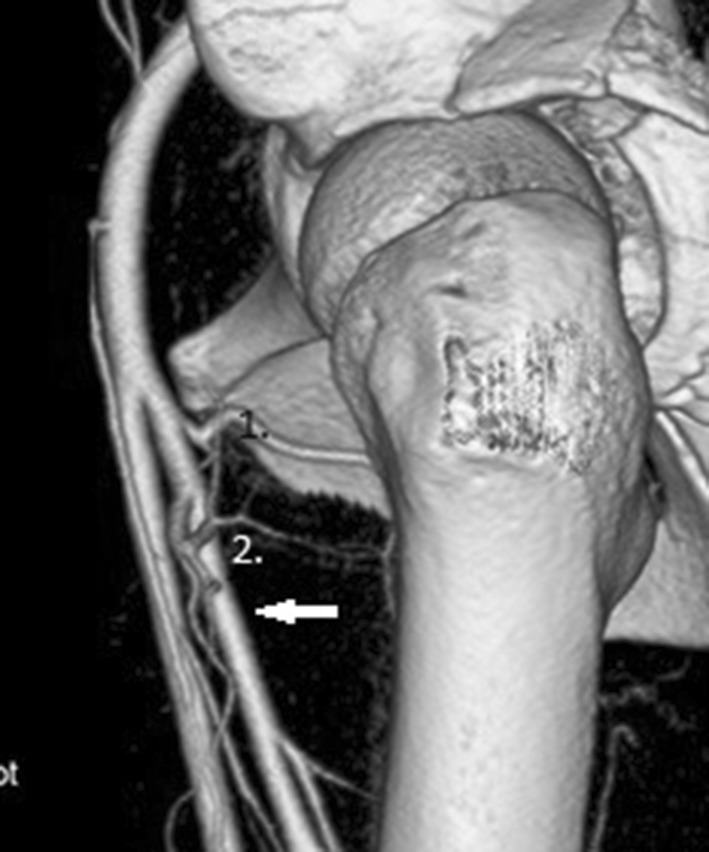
The volume rendering transformation of the angio CT examination of the truncus profundocircumflexus perfectus, MFCA (1), LFCA (2), deep femoral artery (arrow)
